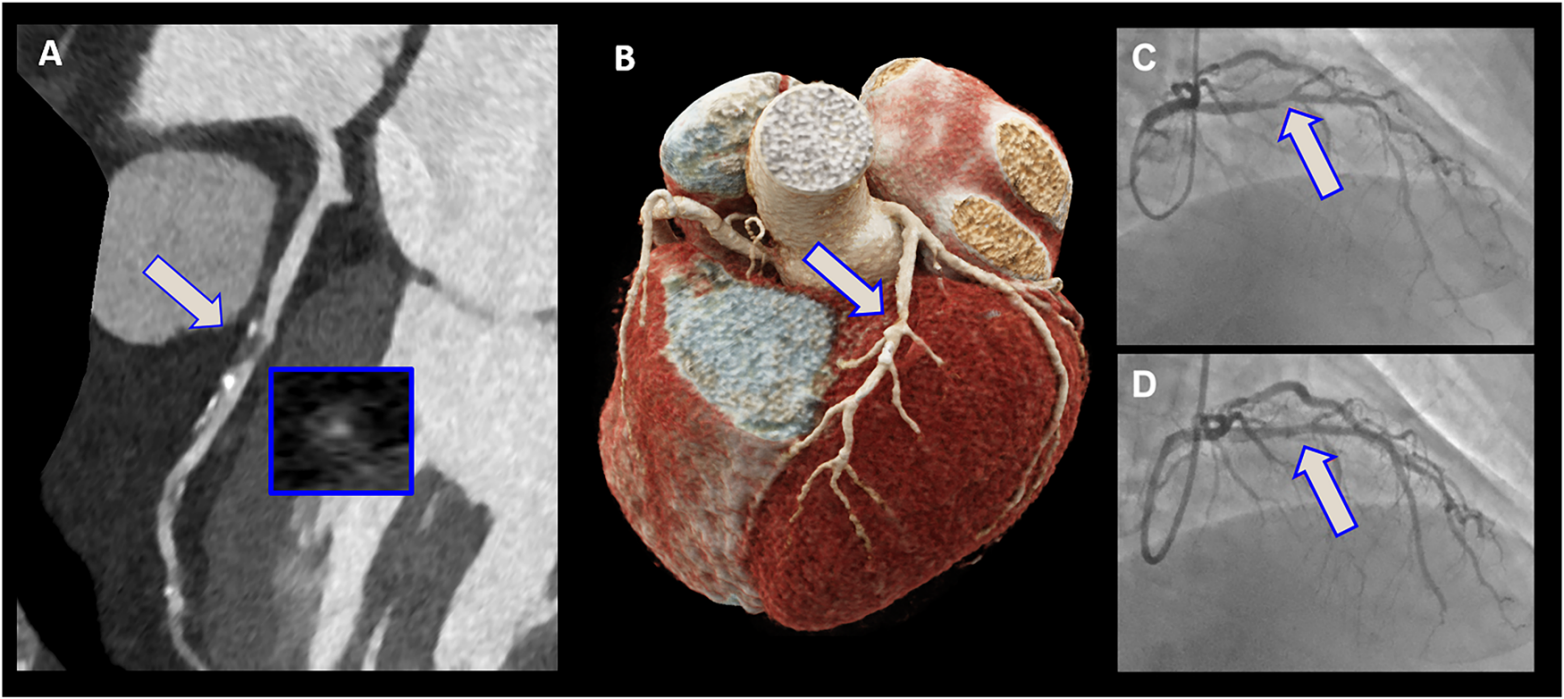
A representative case of PCD-CT for the detection of obstructive CAD. A 72-year-old male with stable chest pain was referred to PCD-CT examination. A ≥ 70% stenosis was detected on the proximal LAD. The patient was then referred to ICA, where a similar stenosis degree on the LAD was found, and PCI was carried out. Panel A shows the multiplanar reconstruction of the LAD, within the small, boxed square, the cross-sectional view can be seen at the stenosis location. B Displays the 3-dimensional view of the heart. C The ICA image of the LAD can be seen with ≥ 70% stenosis in the proximal segment, while D shows the LAD coronary artery after the successful stent implantation. ICA, invasive coronary angiography; PCD-CT, photon-counting detector computed tomography; LAD, left anterior descending coronary artery; CAD, coronary artery disease; PCI, percutaneous coronary intervention
